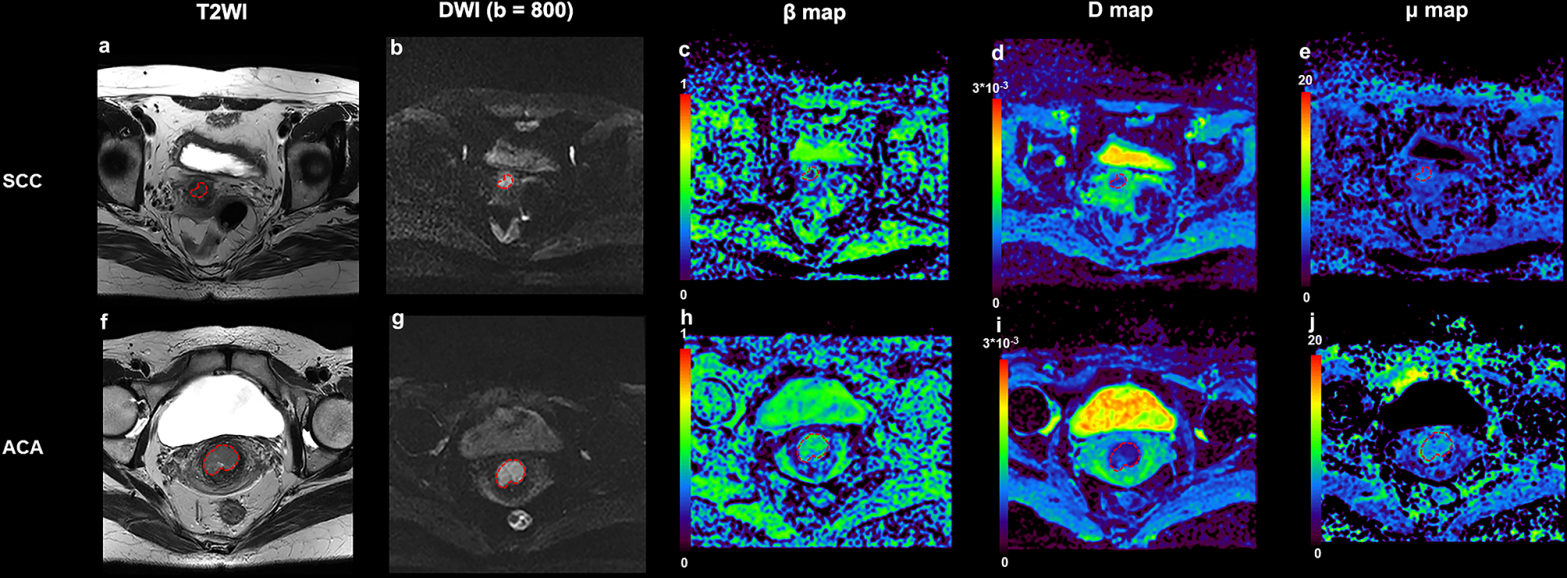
An example of SCC from a 70-year-old patient and ACA from a 56-year-old patient. (A) Transverse T2 image for patient with SCC; (B) DWI image (b = 800 s/mm^2^) for patient with SCC; (C) β map for patient with SCC; (D)
